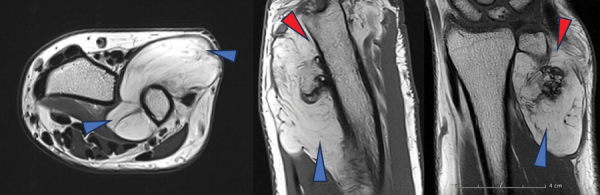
Axial T2-weighted image - Sagittal and Coronal T1-weighted image.
